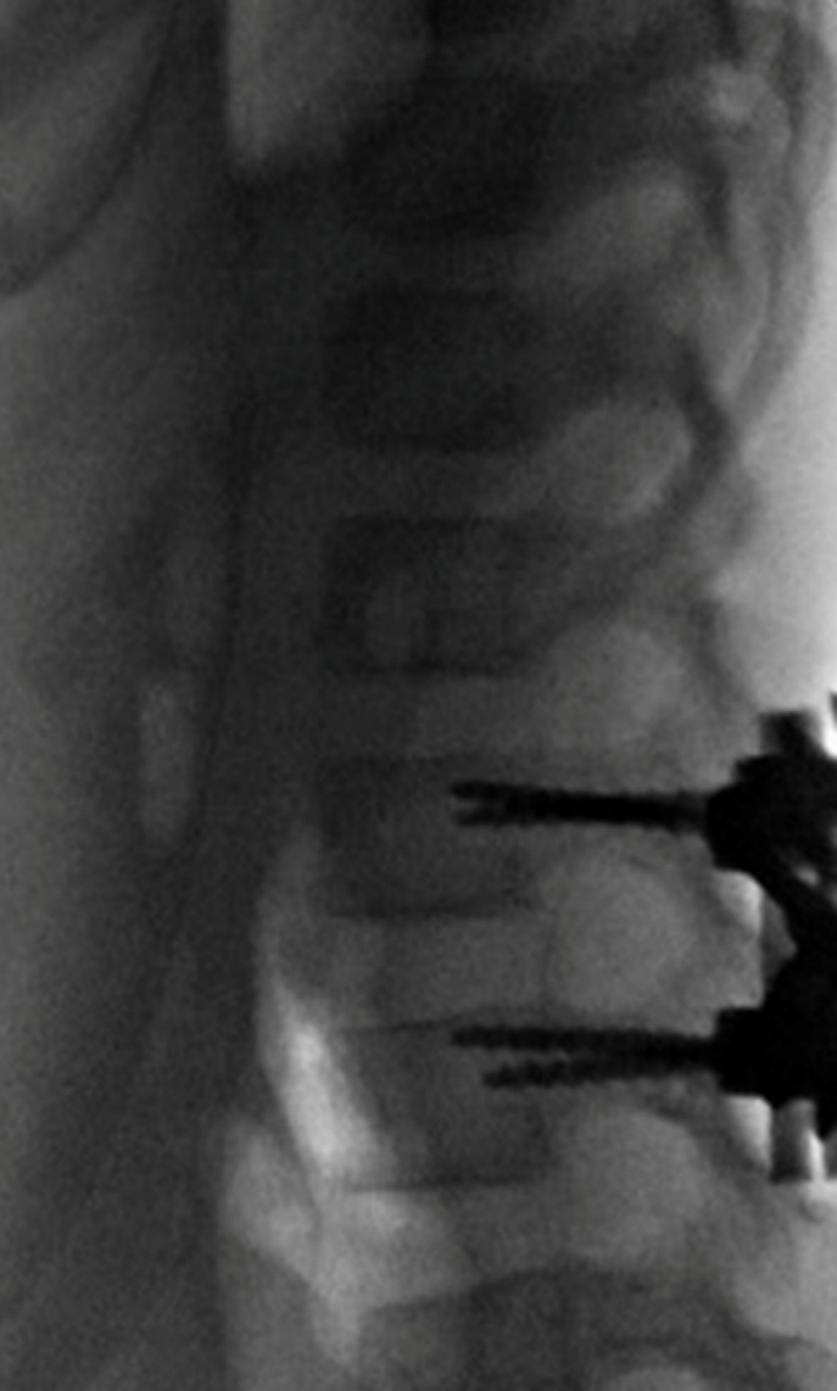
Intraoperative X-ray showing internal fixation and reduction of the facet joint at L2-L3.
